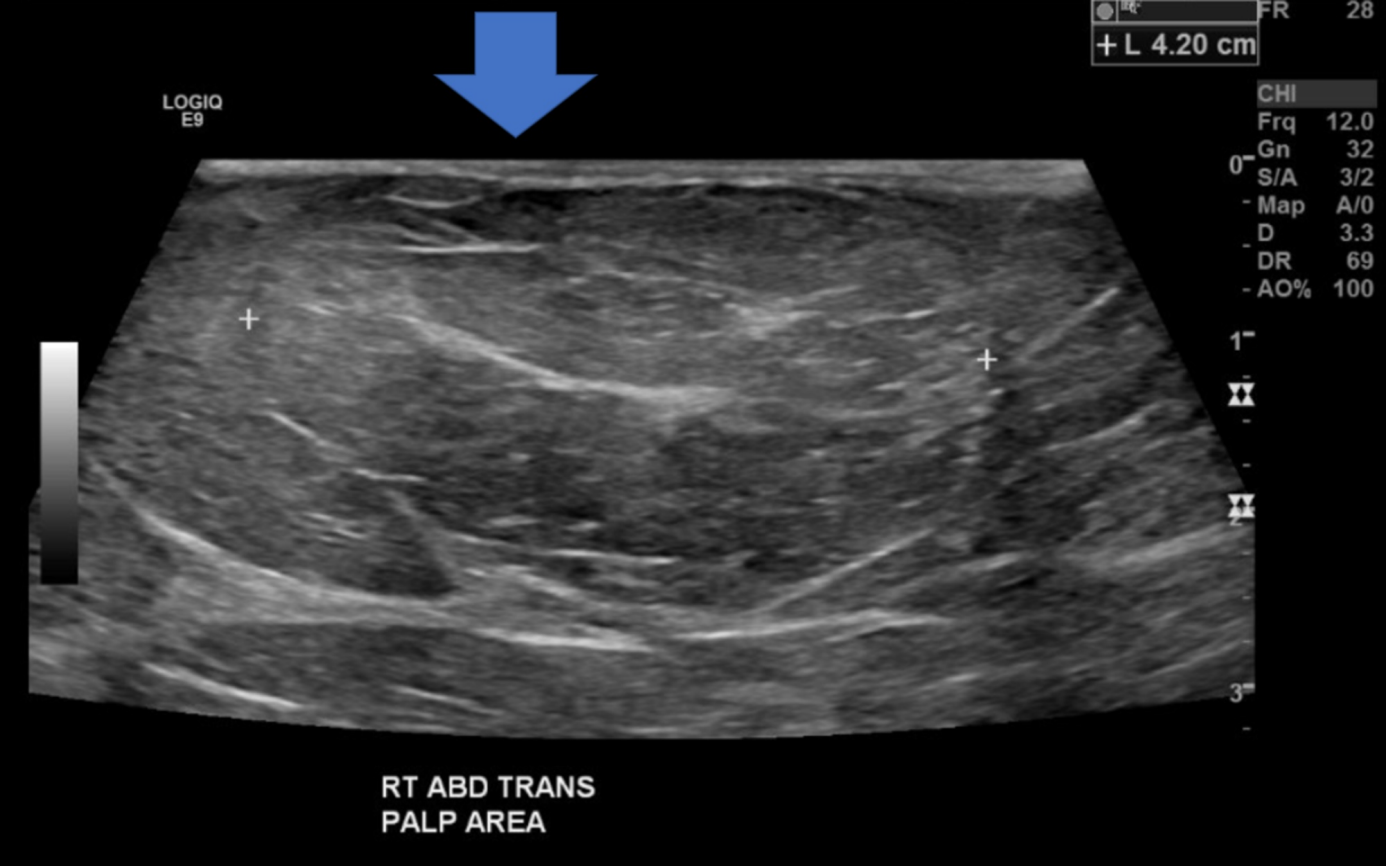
US of abdomen - Suspect fat-containing ventral abdominal wall hernia. US: ultrasound
